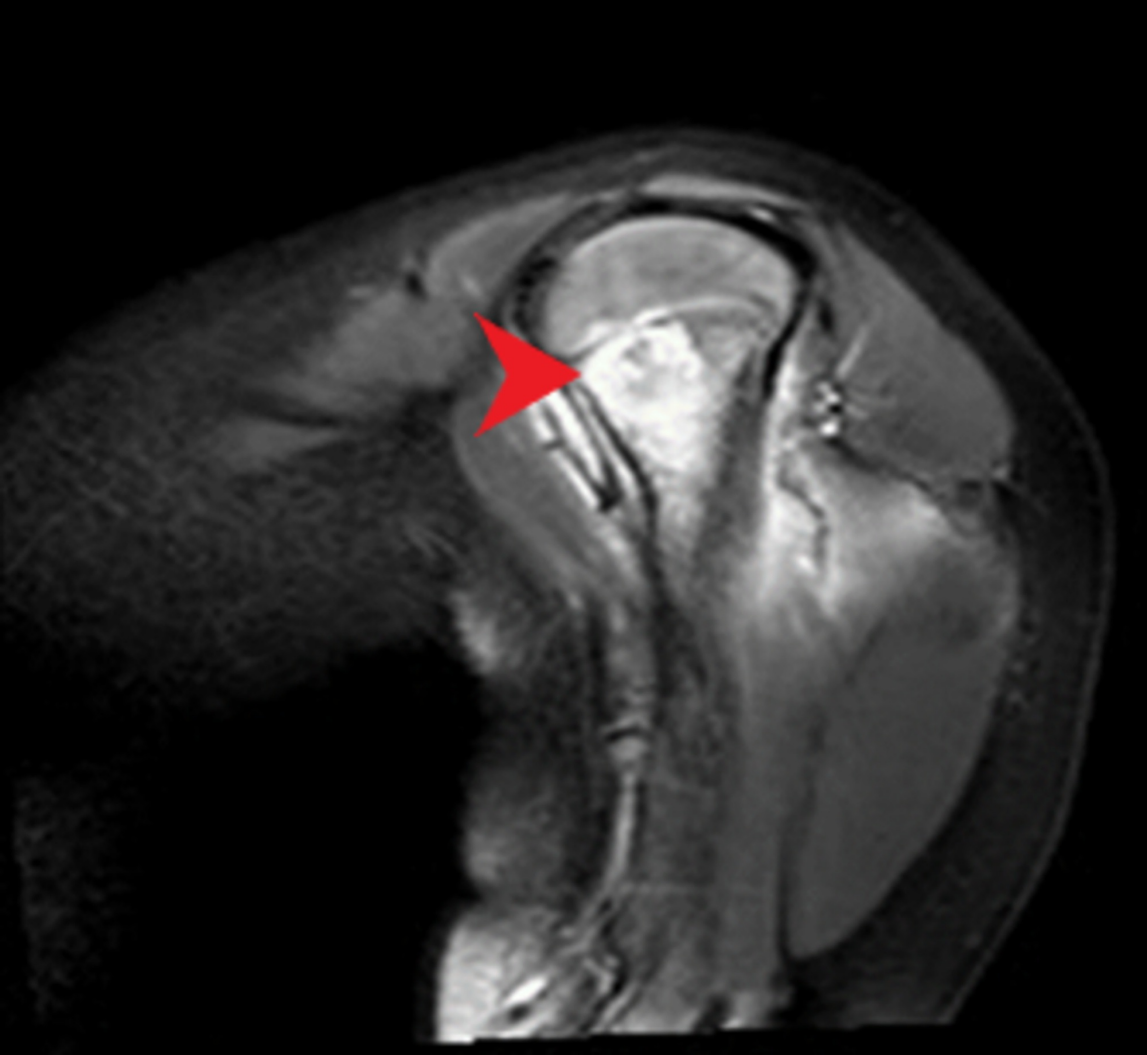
MRI of the proximal humerus showing high signal of the bone and adjacent soft tissues.
